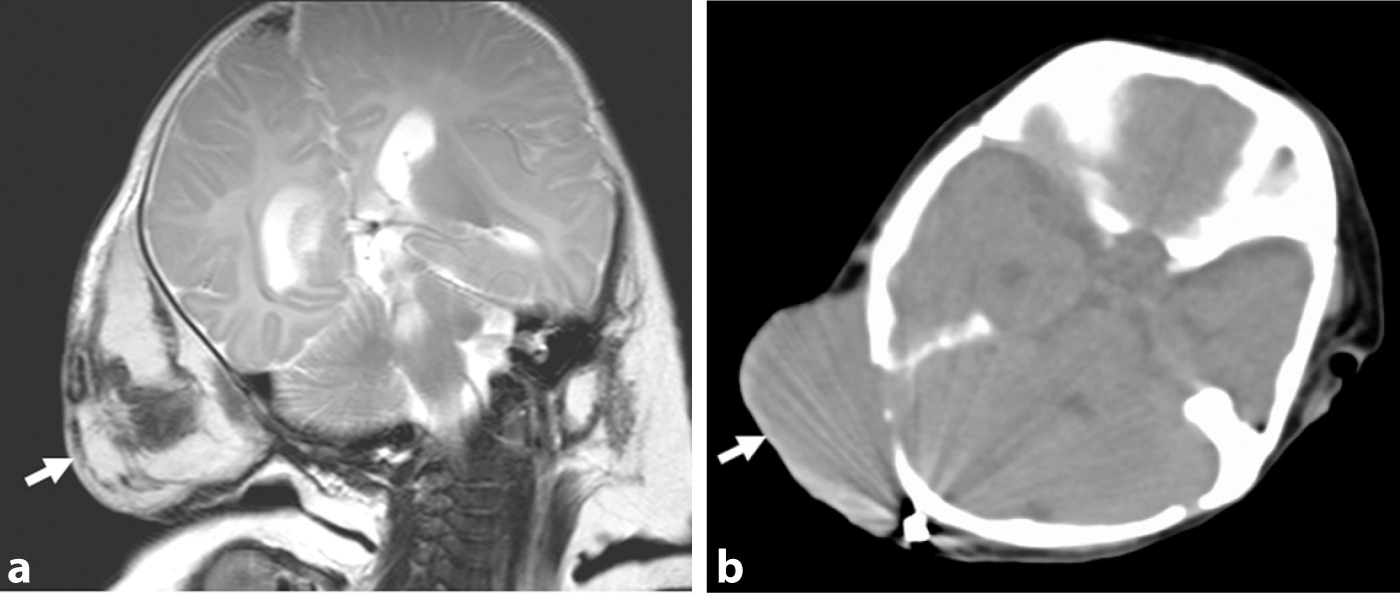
MRI and CT images obtained at the age of 3 months. (a) *T*_2_-weighted MRI showing a large mass with mixed intensity in the right occipital region (arrow). (b) CT scan showing a large mass with bony structure involvement in the right occipital region (arrow).
